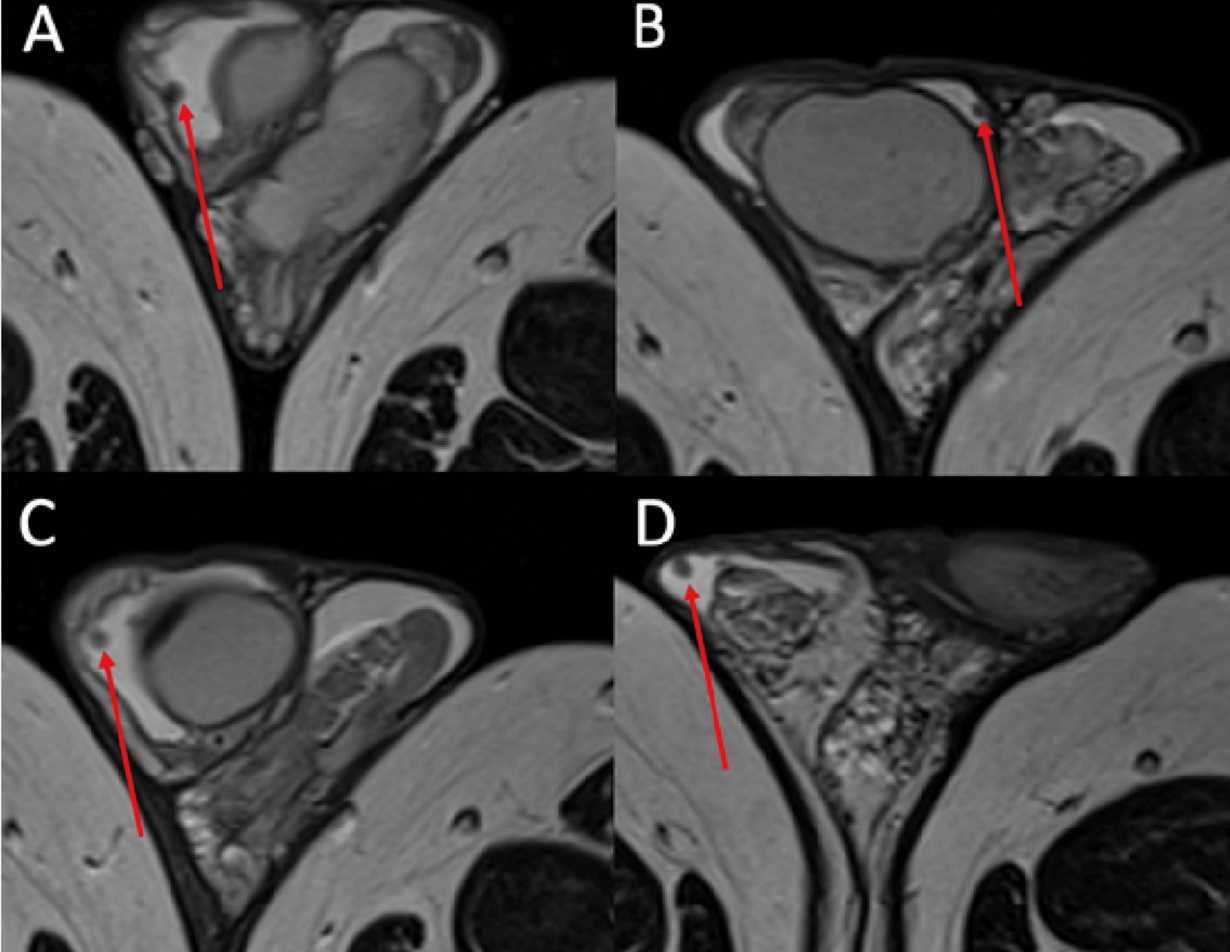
Axial T2-weighted MRI The red arrow points to nodules within the parietal layer of the right tunica vaginalis. These display T2-weighted low intensity in the lateral scrotal wall (A,C), medial wall (B), and anterior scrotum (D).
